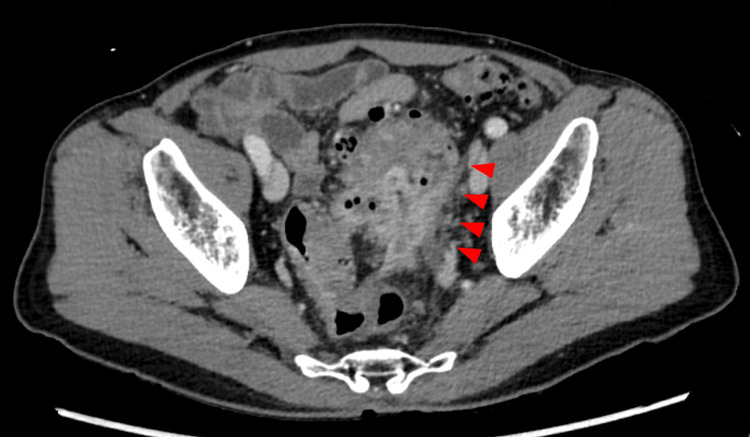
Abdominal computed tomography show that the local advanced tumor was adjacent to the left pelvic wall (red arrowheads)
